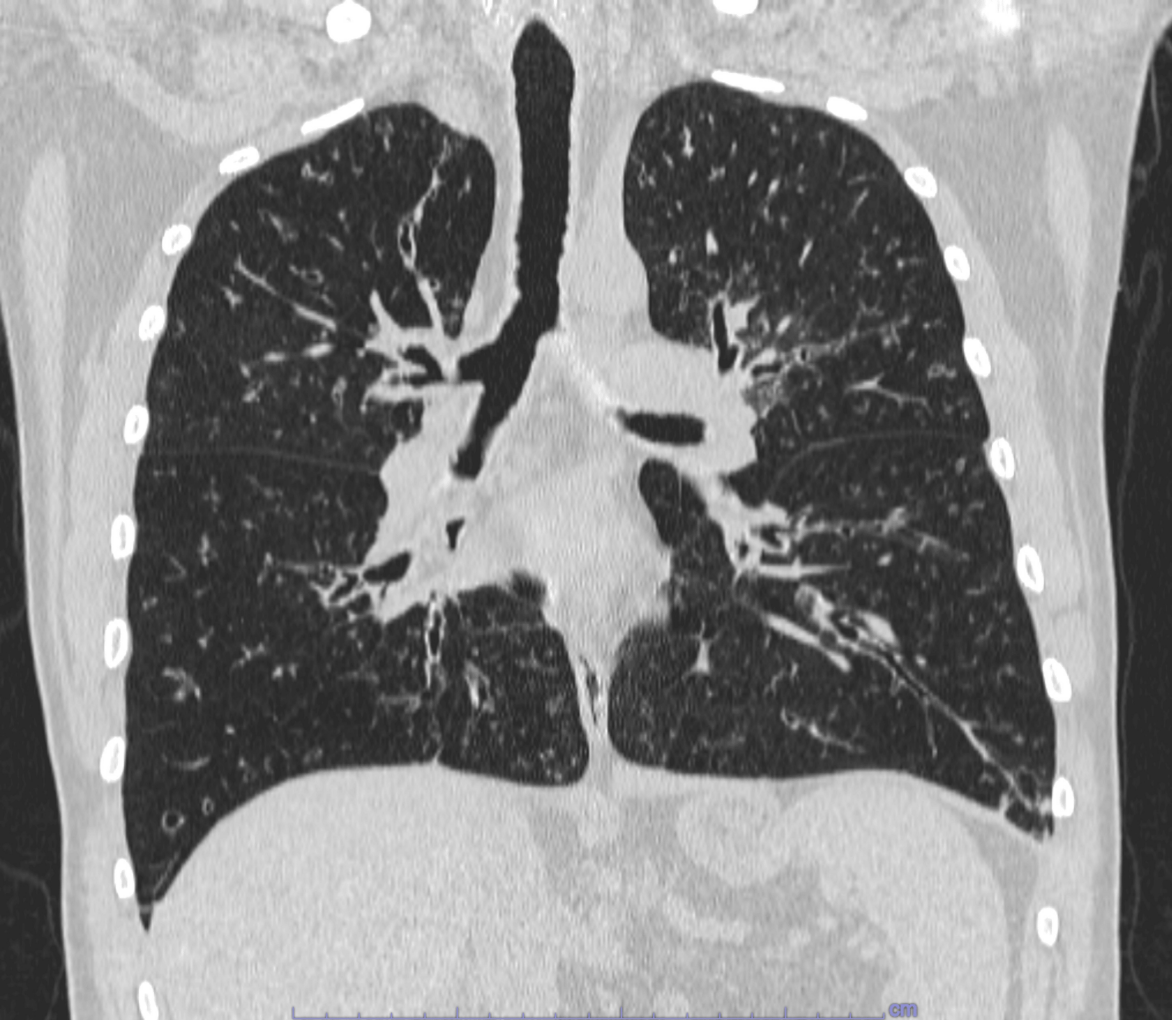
Chest CT scan performed in 2014 The CT shows bilateral cylindrical bronchiectasis and inflammatory involvement of the small airways
